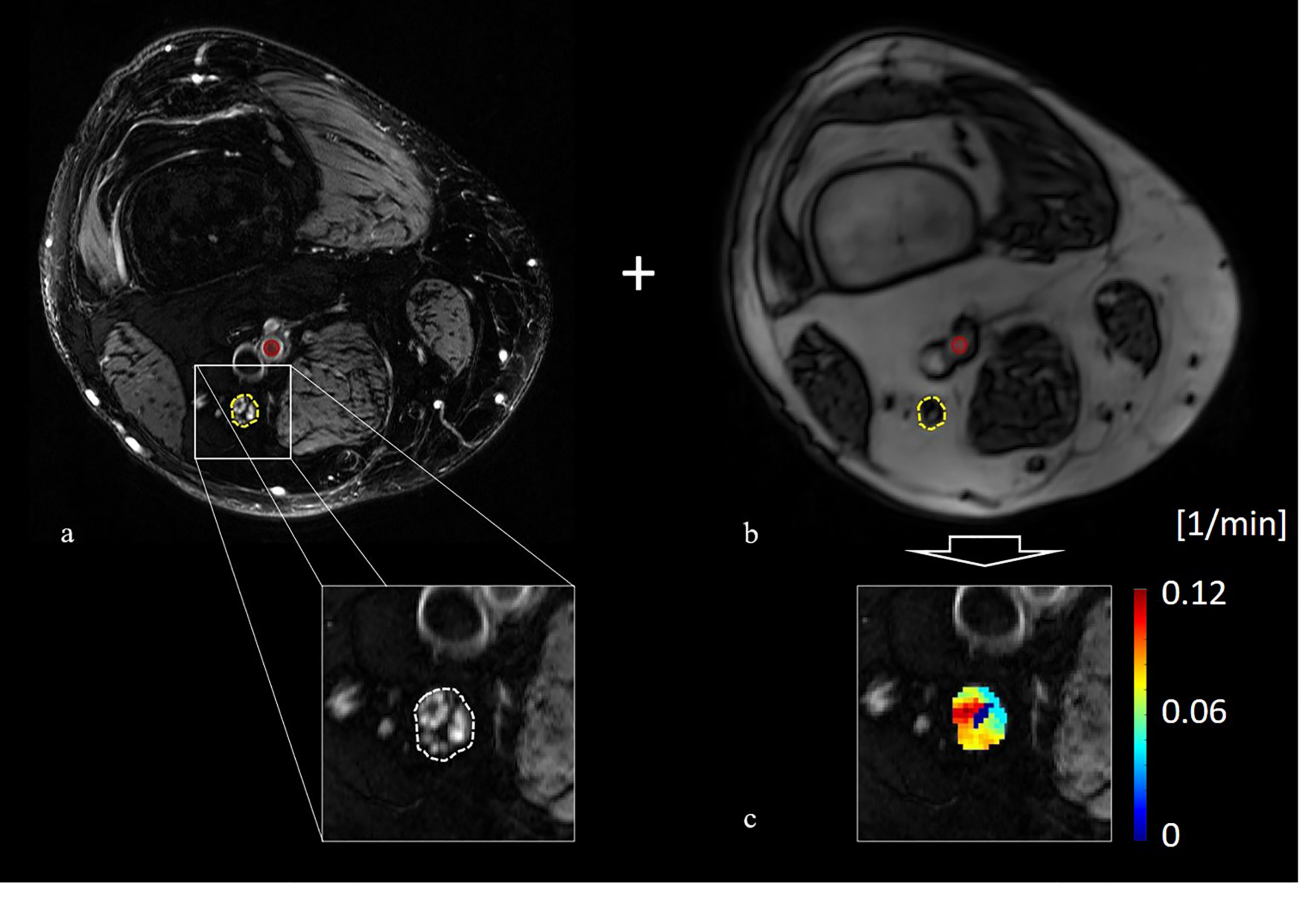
Principle of image co-registration and assessment of nerve perfusion parameters: (A) T2-weighted image of the distal right thigh showing the tibial compartment of the sciatic nerve (yellow circle) and the position of the femoral artery (red circle), (B) axial T1-weighted volume interpolated breathhold examination sequence of the same position a s in (A) with the co-registered position of the sciatic nerve (yellow circle) and the femoral artery (red circle), (C) color coded map of K^trans^ values obtained from the extended Tofts model.
